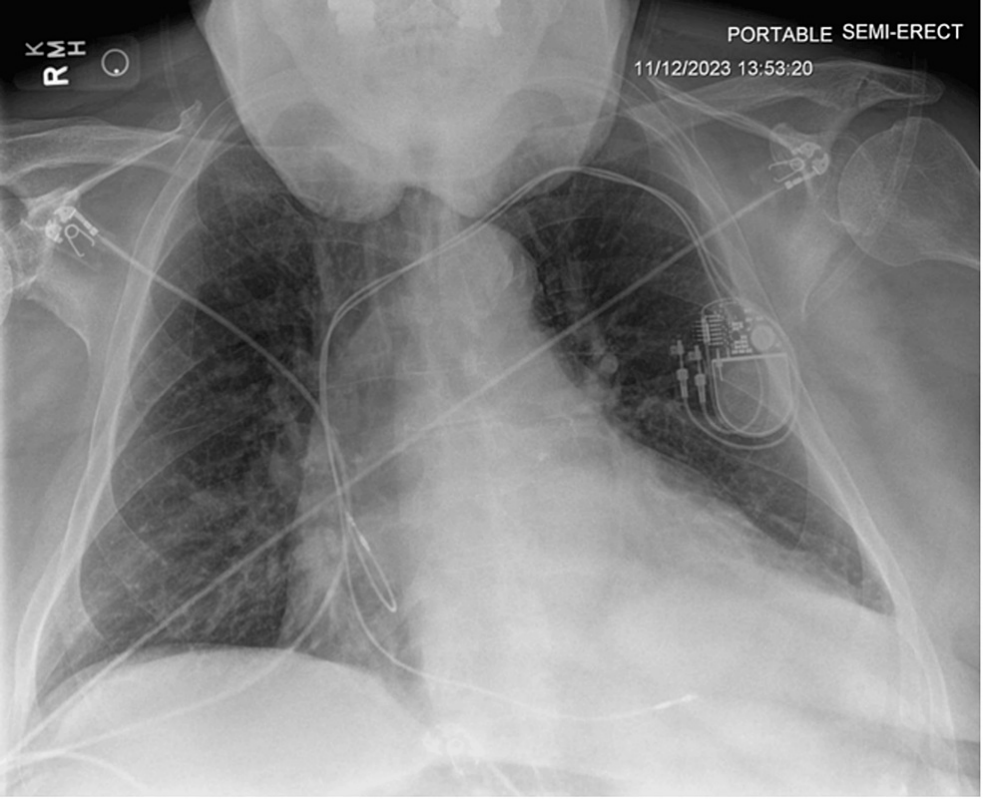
Pre-procedure posteroanterior chest X-ray showing pacemaker position before lead extraction (X-ray obtained on 11/12/2023)
